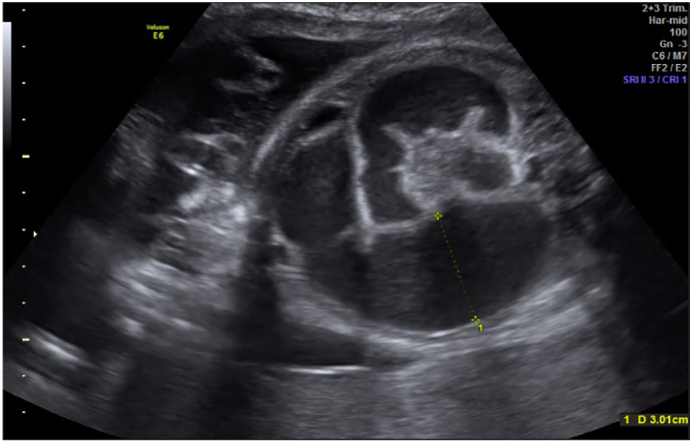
Intestinal atresia identified by prenatal ultrasonography, showing dilation of intestinal loops of 30 mm and interloop edema.
